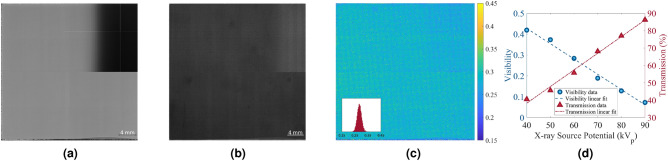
(a) Gain map of the prototype 4K $$\times$$ 4K high-resolution X-ray detector, showing detector defects and artifacts; the top right quarter of the detector is defective. (b) Transmission image for part of the prototype fabricated grating at $$60\,\text{kV}_p$$. (c) Visibility map for the prototype fabricated grating. (d) Visibility and transmission profiles versus X-ray source potential.
